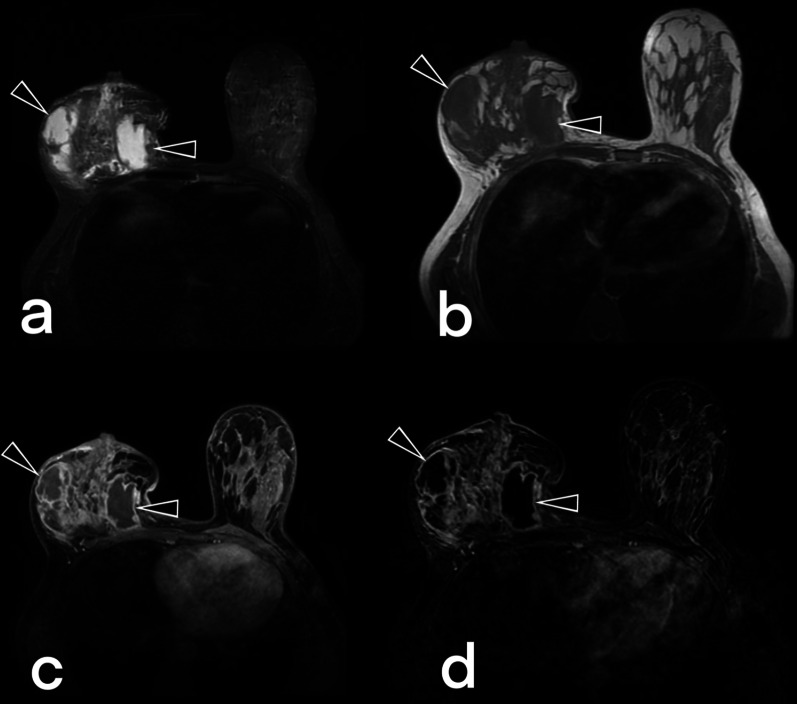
Breast MRI images of a 39-year-old female. T2 weighted fat-suppressed image (a), T1 weighted image (b) post-contrast (c), and subtracted image (d) obtained at the late phase after intravenous gadolinium injection demonstrate multiple fluid collections (arrowheads) with peripheral enhancement in the right breast in keening with abscess. Diffuse involvement. BIRADS 4
